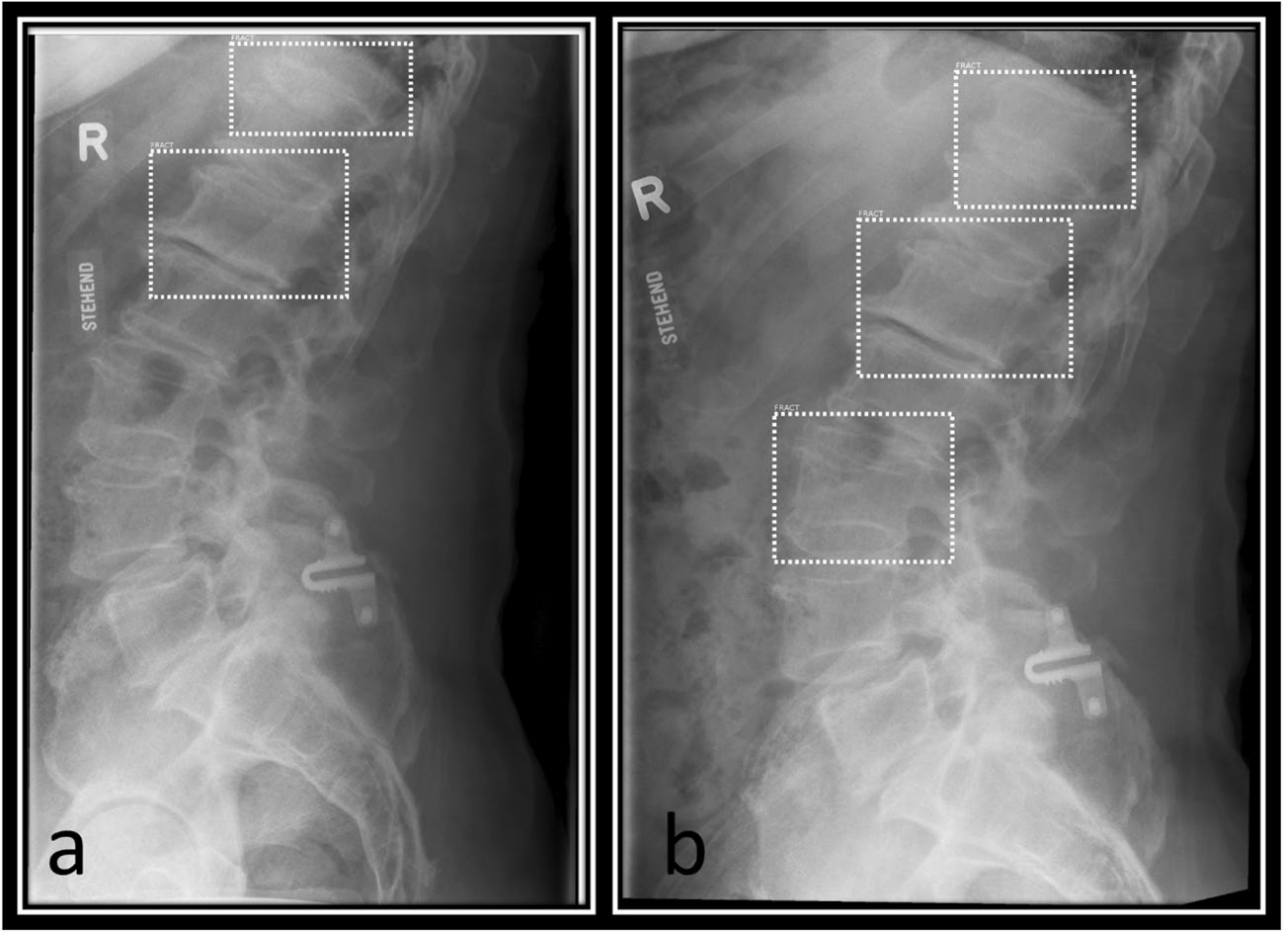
a, b from left to right: lateral spine radiographs of the lumbar spine of an identical patient in ante- and retroflexion with markings by the AI software showing a diversion in results. The AI marks an additional dashed-line bounding box on the L3 vertebra in one image, while only marking L1 and T11 in the other image of the same patient during the same exam, where additional functional imaging was obtained. The fractures in T11 and L1 showed true positive, grade 1 (L1) and grade 2 (T11) compression fractures; L3 shows a false positive
